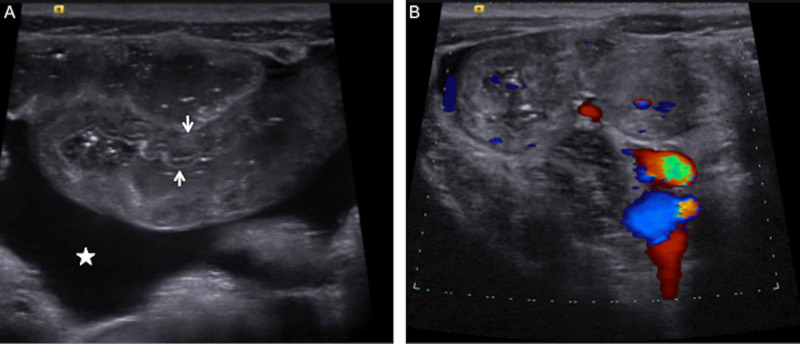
Abdominal Ultrasound A) Lower abdomen midline image demonstrating several loops of small bowel with concentric bowel thickening (between the arrows) and associated simple-appearing ascites (star). B) Right lower quadrant image with color Doppler demonstrating preserved bowel wall flow. Real-time scanning demonstrated no evidence of bowel intussusception or intraperitoneal fluid collection.
